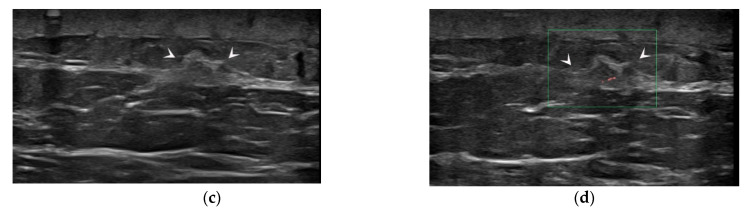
Asymptomatic recurrence after nipple-sparing mastectomy with flap reconstruction. A 42-year-old female patient who underwent nipple-sparing mastectomy with deep inferior epigastric artery perforator flap reconstruction for invasive ductal carcinoma (grade 2, luminal type) 48 months ago. (a,b) Using postoperative MRI as a method of routine postoperative surveillance, an irregularly shaped enhancing focus was noted in the inner region of the left reconstructed breast (arrow). It was a newly noted lesion, according to a comparison with the previous routine surveillance MRI from 12 months earlier. (c,d) Using targeted ultrasound to detect the enhancing lesion, an irregularly shape hyperechoic lesion with indistinct margins confused with the surrounding tissue was noted at the corresponding site (arrow heads). It showed intra-lesional vascularity on the Doppler scan, and it was confirmed as recurrent breast cancer by ultrasound-guided core-needle biopsy.
